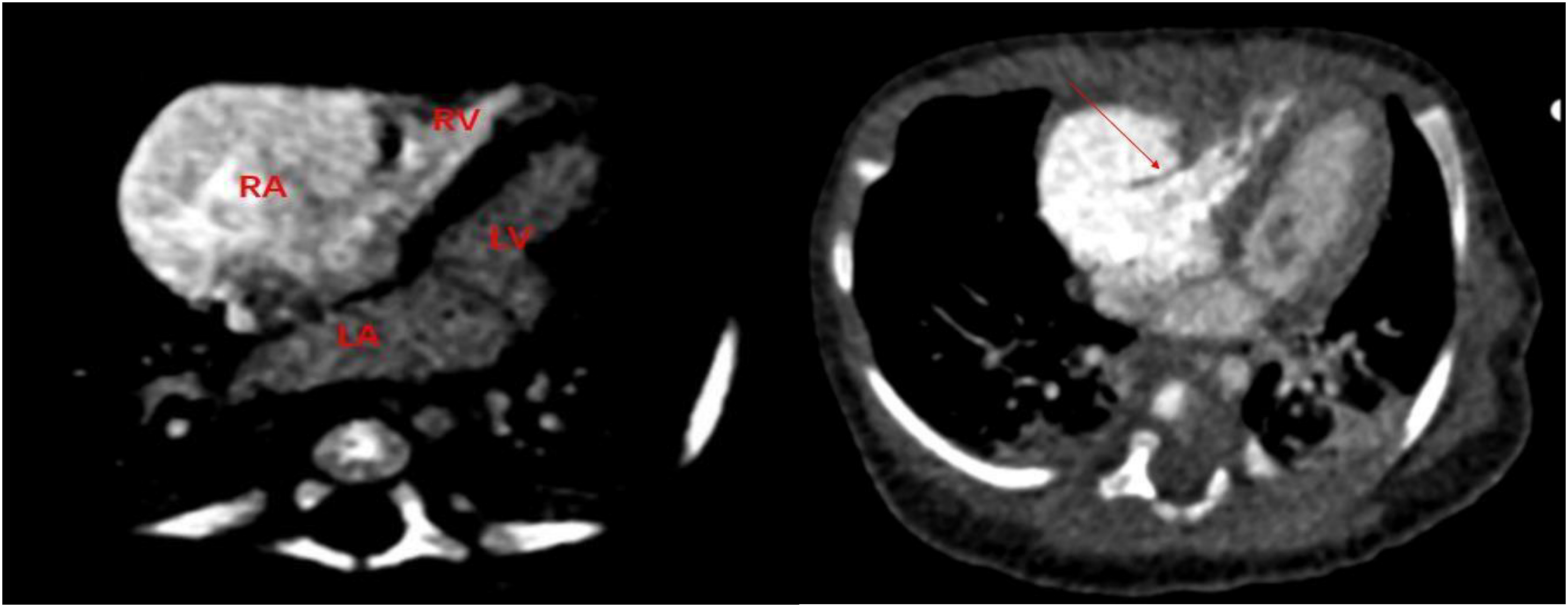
Computed tomography shows the large right atrium and atrial dissection.
